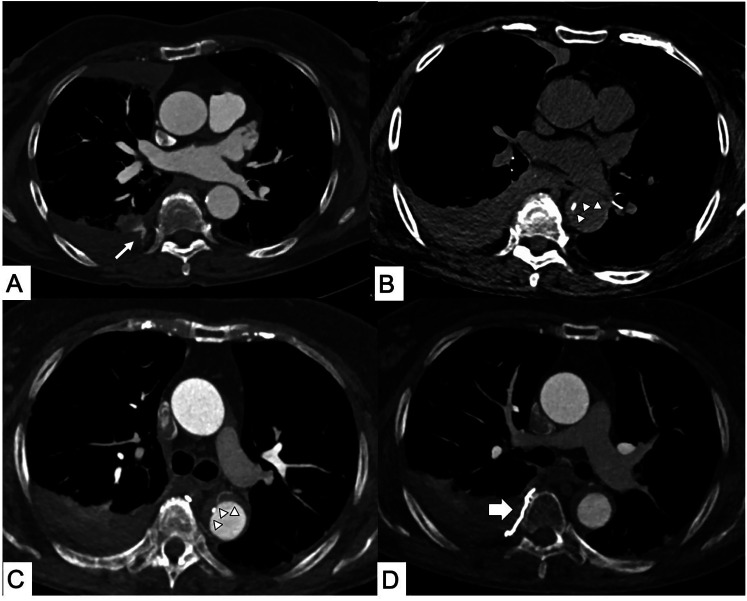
Iatrogenic intramural hematoma (IMH). Computed tomography (CT) axial scans. (A) Right intercostal active bleeding after lung biopsy (thin arrow). (B) Axial CT performed for chest pain 1 day after the intercostal artery transcatheter embolization procedure shows the appearance of hyperattenuated aortic wall thickening from IMH with (C) displacement of intimal calcification and bronchial artery in the contest of the Type B IMH. (D) No more active bleeding in the site of embolization (thick arrow).
